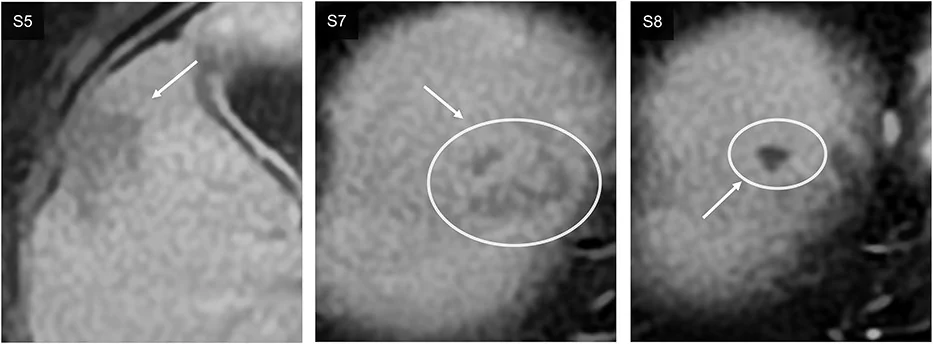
EOB-MRI at 6 months after conventional transarterial chemoembolization, in the arterial phase. Arterial enhancement in the treated segment 5 lesion had resolved, and non-target lesions in segments 7 and 8 became hypovascular.
